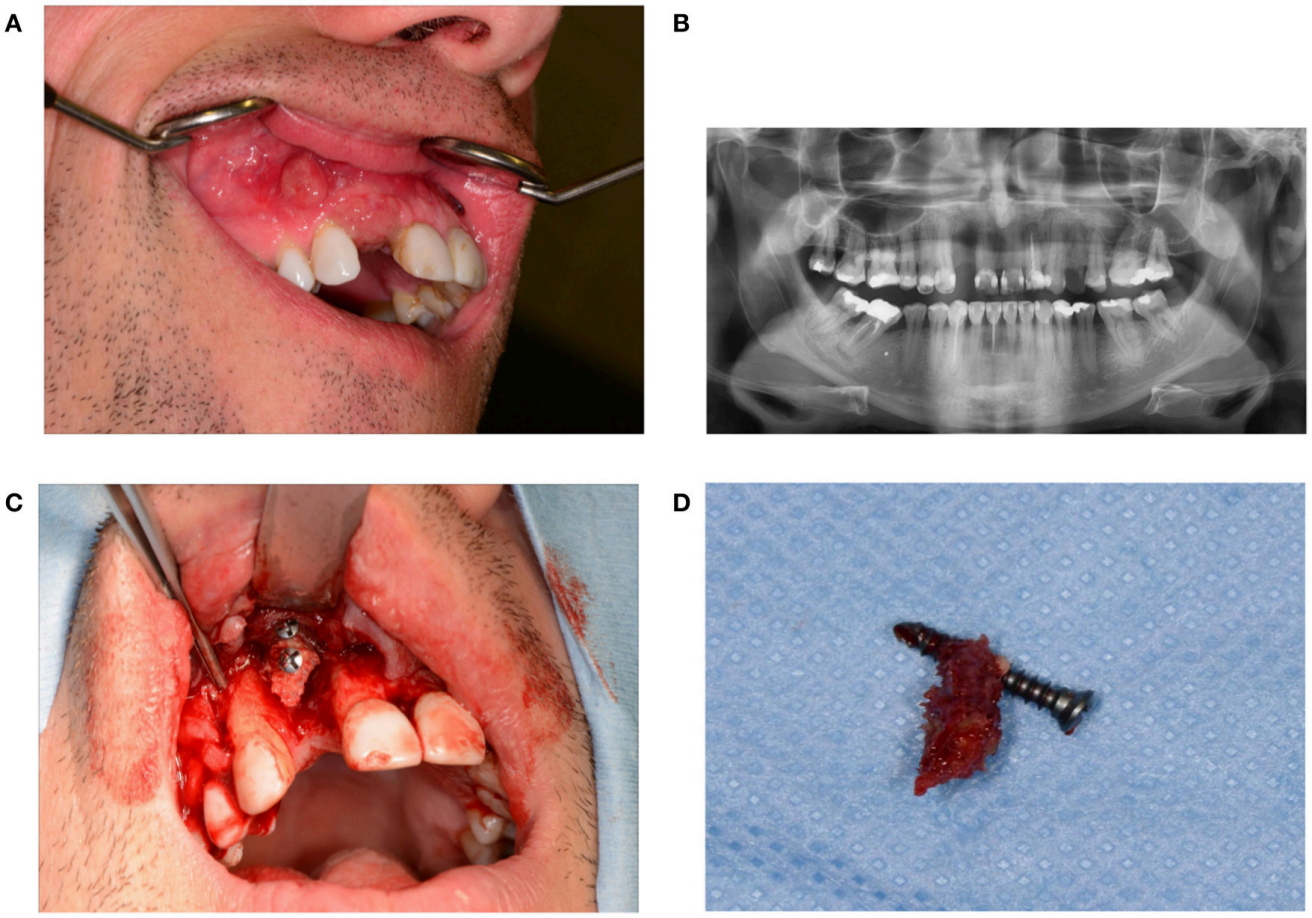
(A) Granuloma formation after reconstruction surgery through a bone from the anterior iliac crest in region 12. (B) Panoramic radiography indicates a vertical bone deficit in region 12. (C) Exploration of a wound from which the residue of a non-healed bone graft was removed. A large part of the originally implanted bone graft has already disappeared due to necrosis and/or resorption. (D) The necrotic bone graft with an osteosynthesis screw.
